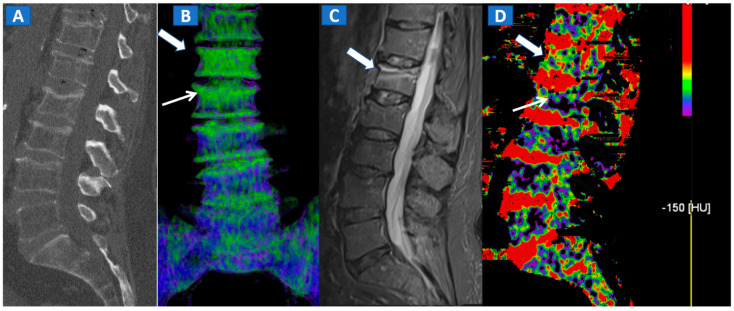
A 62-year-old female with traumatic low back pain following a fall. On the sagittal standard 1 mm CT image (A) it is not possible to identify the fresh fracture. On the coronal 3D DECT image (B), BME of the L1 vertebral body is coded in green (thick arrow), with some mild oedema depicted on the L2 superior endplate (thin arrow). On the sagittal STIR MRI image (C), a fresh fracture of the L1 body is confirmed, with mild oedema located close to the superior endplate (thick arrow). There is no BME of the L2 body apparent on the STIR MRI image. On the corresponding 2D DECT image (D), the maximum level of superimposition of color-coded maps was used to confirm the presence of mild oedema on the L1 vertebral body (thick arrow) and to rule out the presence of significant oedema on the L2 body (thin arrow), thus avoiding a false positive finding.
